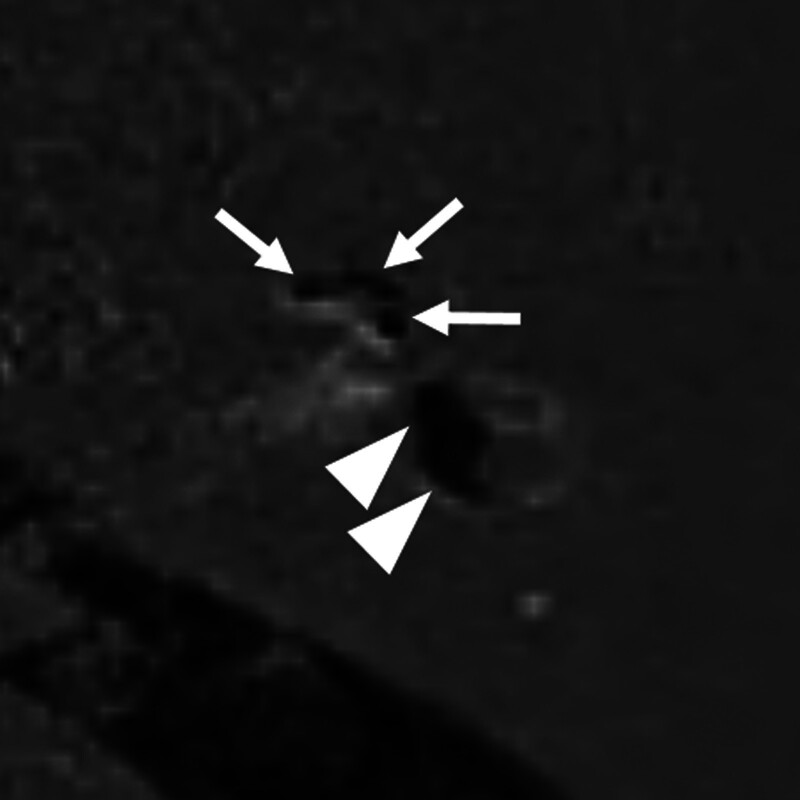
Inner ear magnetic resonance imaging (MRI) of a 45-year-old male with left sudden sensorineural hearing loss. Grade II cochlear hydrops and grade III vestibular hydrops are detected on a 0.1 mm axial hybrid of reversed image of positive endolymph signal and native image of positive perilymph signal (HYDROPS) image of inner ear MRI, in which a fully obliterated scala vestibuli (arrows) and a complete confluence of saccule and utricle (arrowheads) without a distinct enhancing perilymphatic rim are visible.
